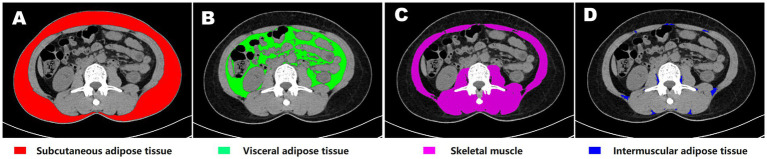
Representative CT images of body composition semi-automated segmentation at the third lumbar vertebra level. Four core body composition compartments were segmented based on specific Hounsfield unit thresholds: (A) Subcutaneous adipose tissue (red); (B) Visceral adipose tissue (green); (C) Skeletal muscle (purple); and (D) Intermuscular adipose tissue (blue).
